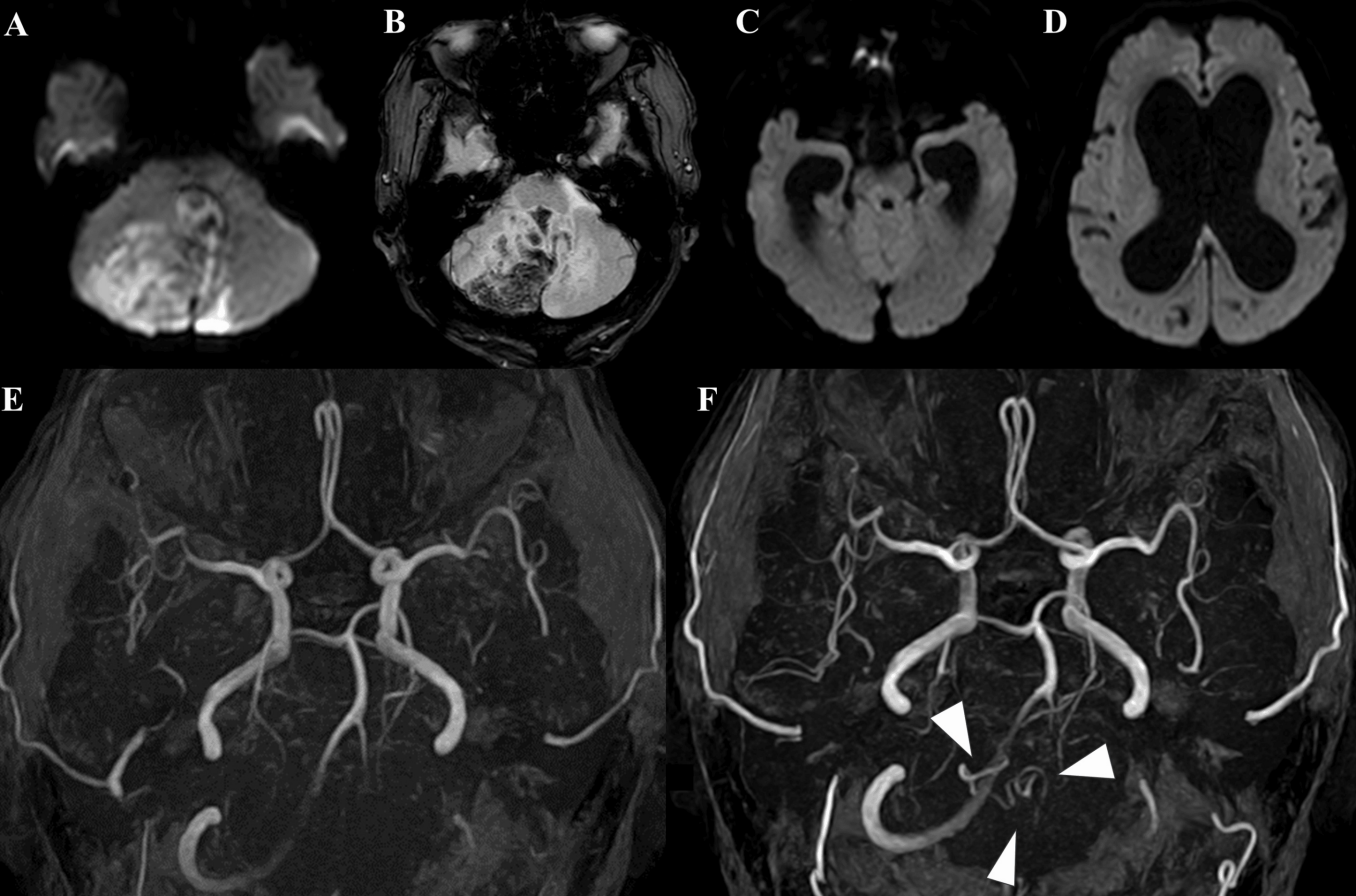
MRI and MRA in a patient with bilateral PICA infarction (A) DWI shows bilateral cerebellar infarctions sparing the brainstem. (B) T2*-weighted imaging shows low signal intensity in the infarcted areas. (C, D) DWI shows ventricular enlargement, consistent with obstructive hydrocephalus. (E) MRA from the current presentation shows occlusion of the right PICA. (F) MRA performed two years earlier reveals a bihemispheric PICA variant arising from the right vertebral artery, while the contralateral PICA was not visualized (arrowhead indicates the right PICA). DWI, diffusion-weighted imaging; MRA, magnetic resonance angiography; PICA, posterior inferior cerebellar artery
